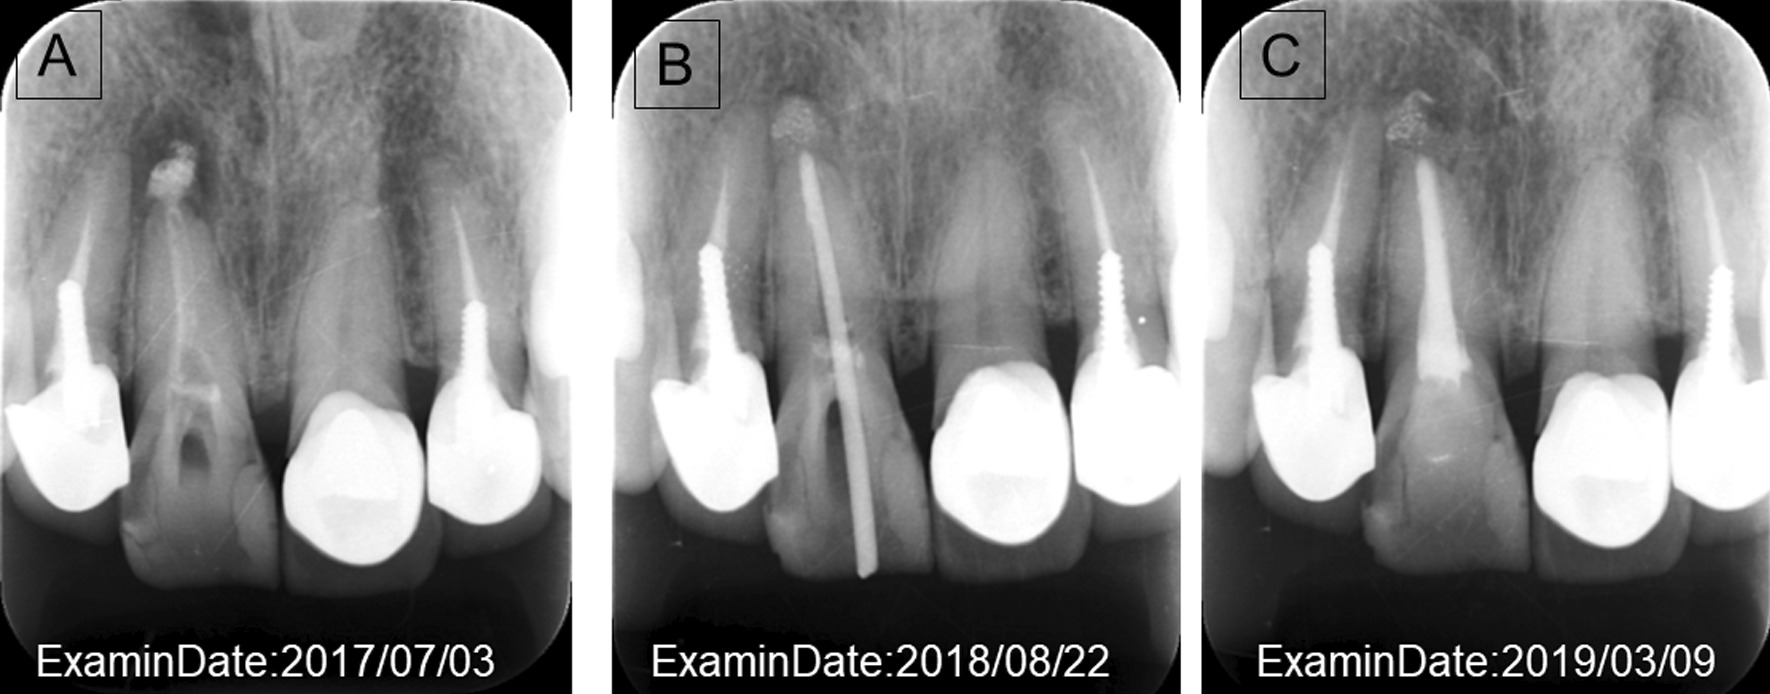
a Radiograph during follow-up on 3 July 2017. This image shows that there is a cyst in the tooth root. b Radiograph of root canal treatment 22 August 2018. Root canal treatment was initiated; the root canal was thoroughly cleaned and disinfected, then filled with medication and covered; it was closely monitored during follow-up. c Radiographs after treatment on 9 March 2019. The root cyst in the affected area improved, and the patient’s pain disappeared
